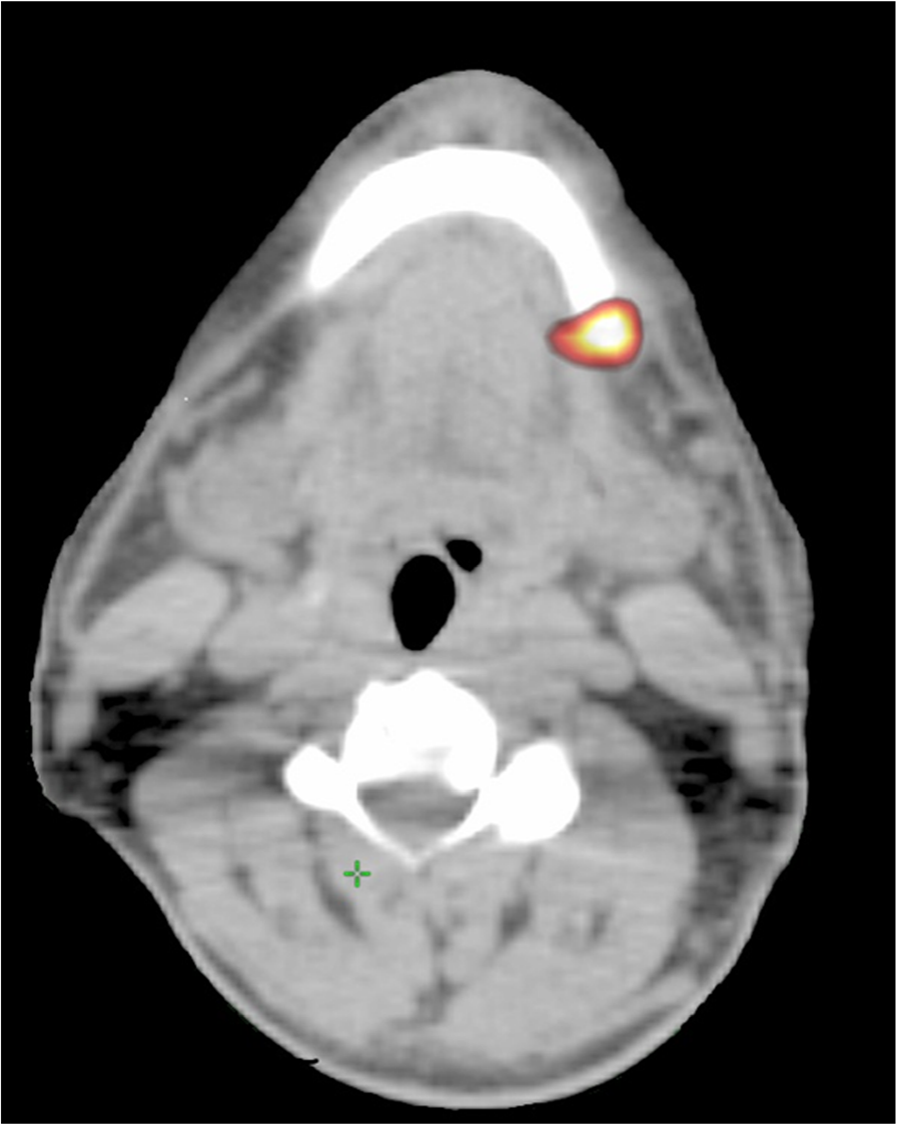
A positron emission tomography/computed tomography scan identified increased fluorodeoxyglucose uptake in the mandible bone.
